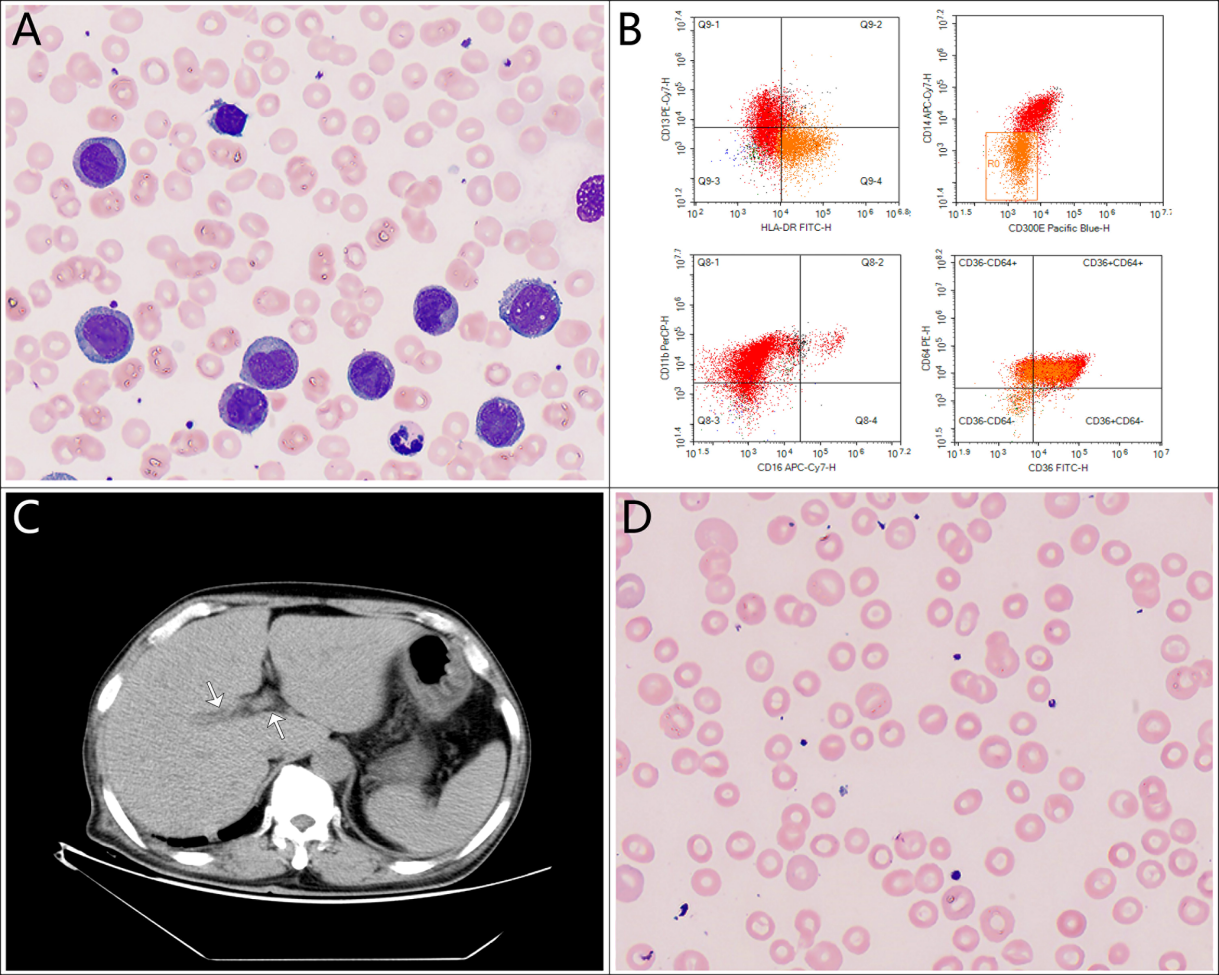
Key diagnostic findings in this case. (A) Peripheral blood smear showing a significant number of immature monocytes; (B) Bone marrow flow cytometry confirming HLA-DR^+^CD14^-^CD300e^-^ immature monocytes; (C) Abdominal CT scan showing edema of Glisson’s capsule; (D) Peripheral blood smear with target cells.
